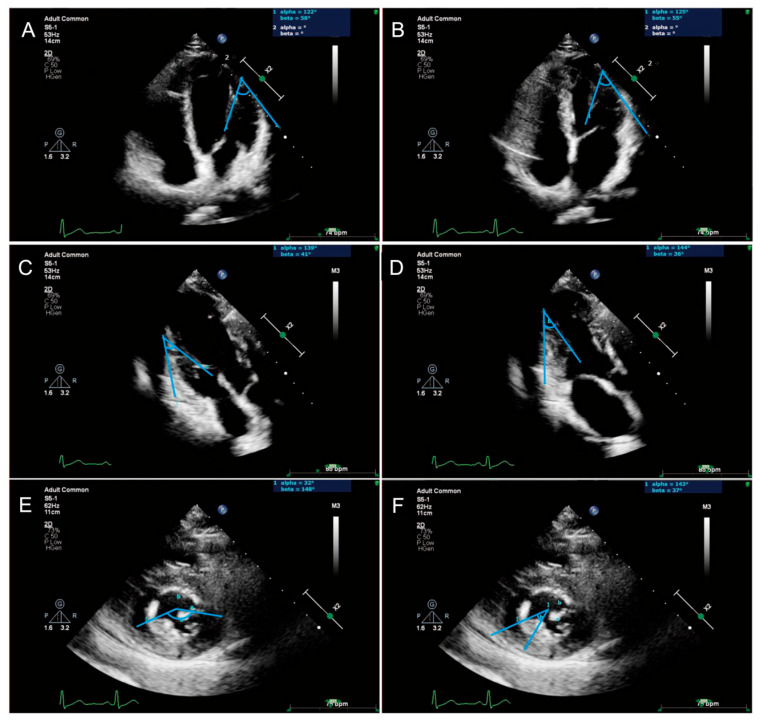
Representative echocardiographic images for left ventricular papillary muscle measurements. (A,B) Diastolic and systolic anterolateral papillary muscle (APM) with left ventricular wall angle; (C,D) diastolic and systolic posteromedial papillary muscle (PPM) with left ventricular wall angle; (E,F) systolic circumference angle of APM and PPM. APM, anterolateral papillary muscle; PPM, posteromedial papillary muscle.
